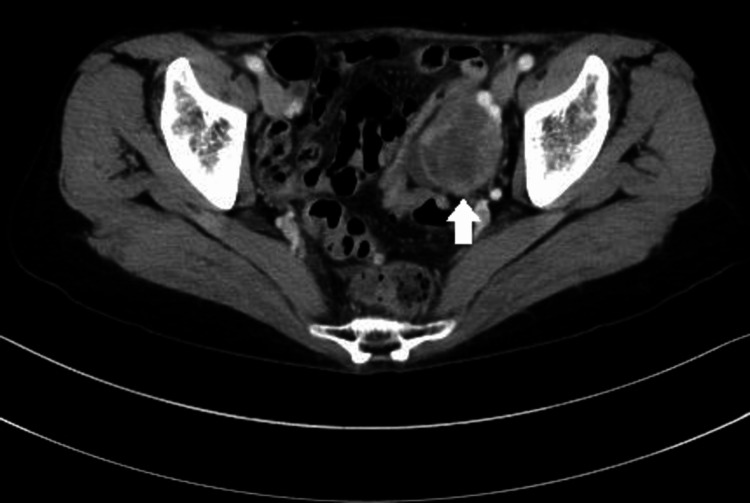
Contrast-enhanced pelvic CT image. A solid tumor, approximately 45 mm in size was observed in the left ovary (arrow).
